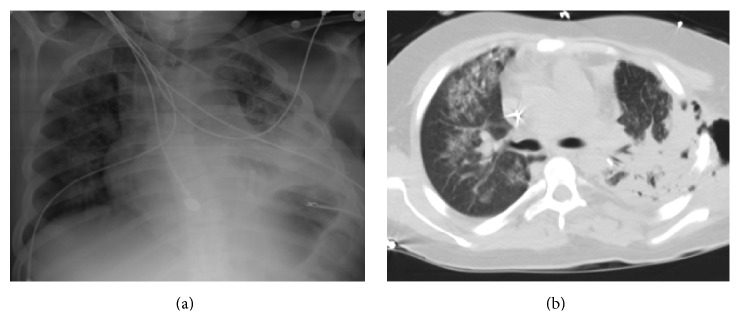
18-year-old male renal transplant recipient 24 days status after resection of left lower lobe for a fungating mass with (a) chest X-ray demonstrating left upper lobe consolidation and (b) chest computerized topography scan showing consolidation of right upper, middle, and lower lobes.
